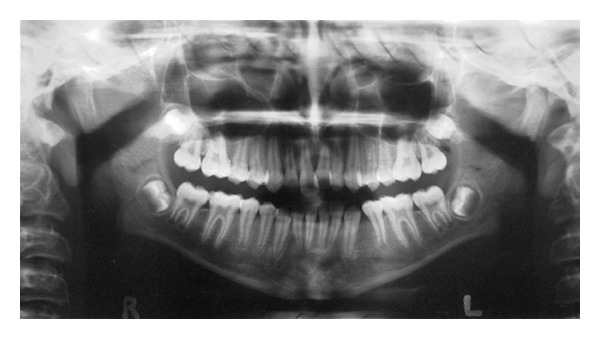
Panoramic radiograph of the same patient as in Figure 5 at 13 years of age showing no sign of the supernumerary tooth.
